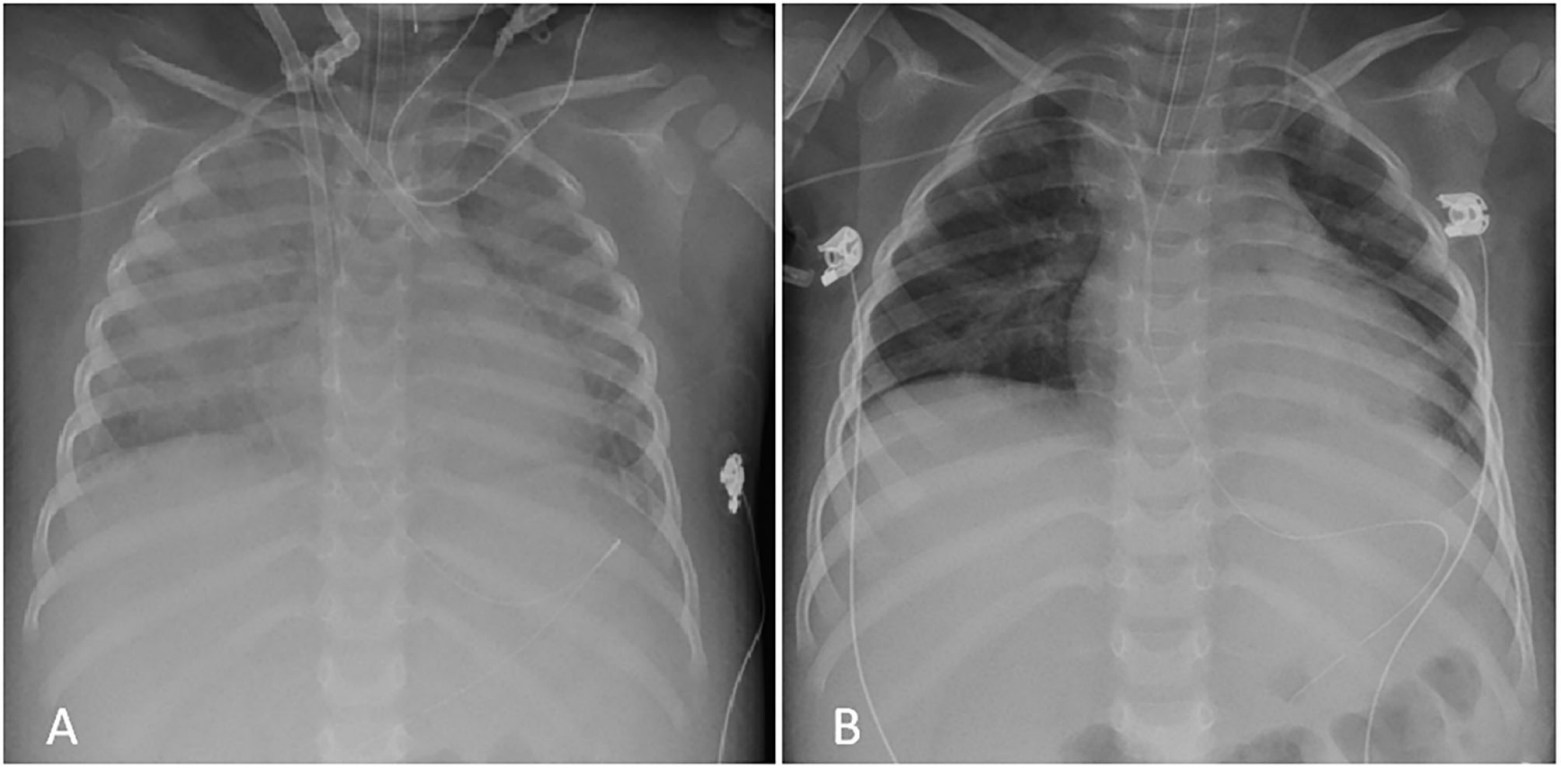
Chest X-Ray during PICU admission. (A) Severe bilateral interstitial thickening and right perihilar consolidation soon after VA-ECMO placement (day 4); (B) Complete resolution of the pattern after HDIV-ANA treatment at PICU discharge (day 16).
